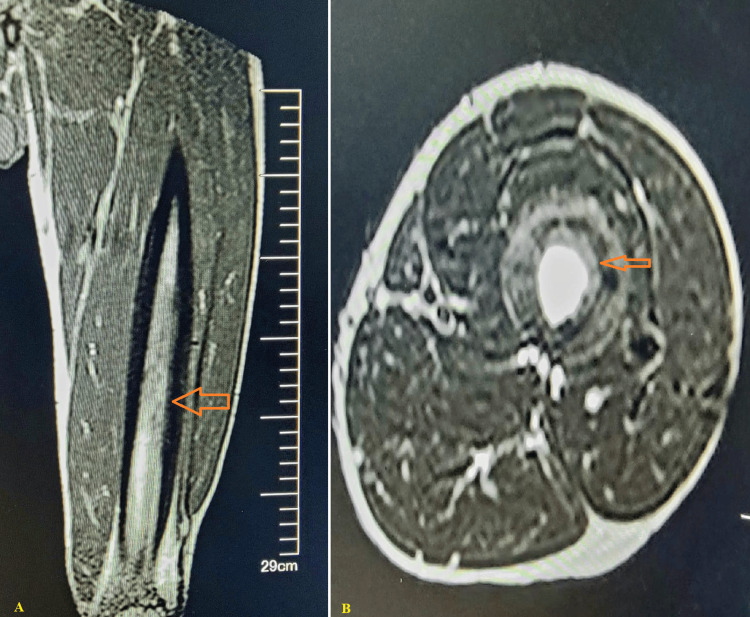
MRI of the left femur Sagittal T1-weighted (A) and axial T2-weighted (B) sequences. (A) An area of hypointense marrow signal (darkened area) within the medullary canal (orange arrow). (B) A corresponding focus of marked hyperintense signal (bright white area) (orange arrow), consistent with fluid collection or pus within an intraosseous abscess (Brodie’s abscess). The surrounding hyperintensity in the vastus musculature represents moderate reactive soft tissue edema, a common inflammatory response to underlying bone infection. MRI: magnetic resonance imaging
